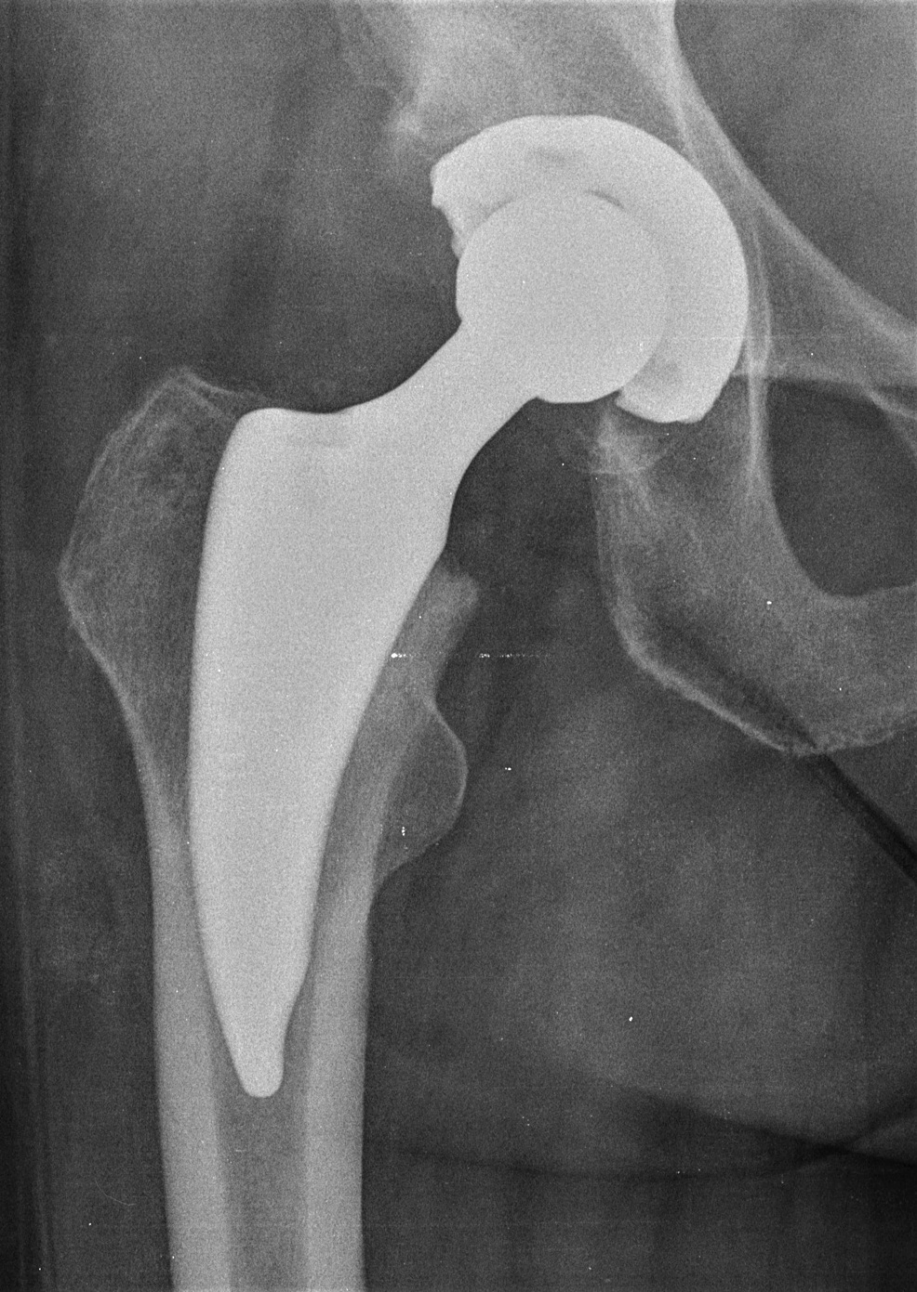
Post-operative x-ray of the MINIMA® short stem
